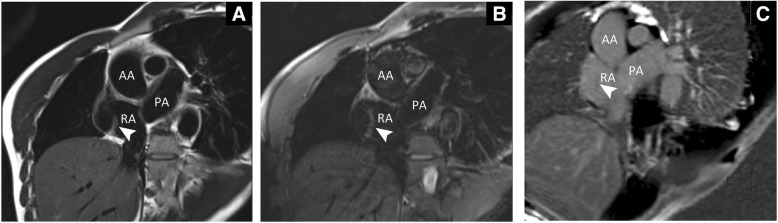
Short axis view. a. On T2-STIR image, the mass (arrowhead) had similar signal intensity to myocardium revealing a myocardial structure and excluding lipoma. b. On T2-STIR with fat suppression image, the consequence is consistent with (a). c. LGE-sequences indicated no abnormal enhancement of the mass (arrowhead). T2-STIR, T2-weighted short tau inversion recovery; RA, right atrium; PA, pulmonary artery; AA, ascending aorta; LGE, late gadolinium enhancement
